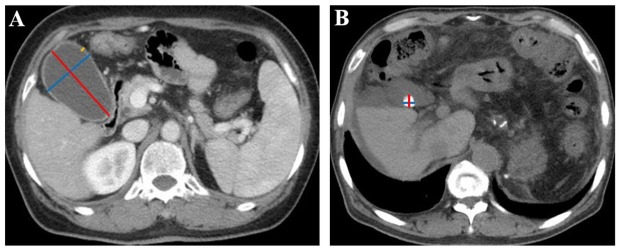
Gallbladder and gallstone parameters measured in CT images. (A) Measurement of gallbladder parameters in a 63-year-old woman with liver cirrhosis. The red line represents the maximum gallbladder length, the blue line represents the maximum gallbladder width, and the yellow line represents the maximum gallbladder wall thickness. The maximum gallbladder length was 77.6 mm, the maximum gallbladder width was 49.0 mm, and the maximum gallbladder wall thickness was 3.5 mm. (B) Measurement of gallstone parameters in a 65-year-old man with liver cirrhosis. The red line represents the maximum gallstone length, and the blue line represents the maximum gallstone width. The maximum gallstone length was 12.7 mm, and the maximum gallstone width was 12.4 mm. CT: computed tomography.
